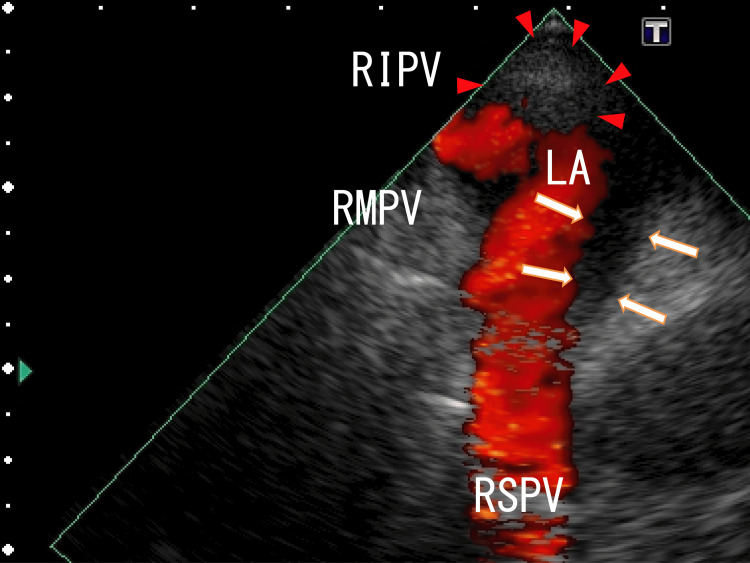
TEE images obtained after one month of standard-dose heparin-warfarin remedy showing partially resolved thrombi TEE images obtained after one month of standard-dose heparin-warfarin remedy showing partially resolved thrombi in the RSPV and LA that appeared to be linked to the thrombi in the RSPV (arrows). The thrombi included no white areas. Thrombi expanded from the RIPV and were depicted as dim white areas (arrowheads). Blood flow from the RSPV and RMPV were shown as red areas. The blood flow from the RIPV could not be detected; however, white LA thrombi were observed in front of the exit of the RIPV. The thrombi were likely the expanded LA thrombi from the RIPV thrombi. LA, left atrium; RIPV, right inferior pulmonary vein; RMPV, right middle pulmonary vein; RSPV, right superior pulmonary vein; TEE, transesophageal echocardiography
